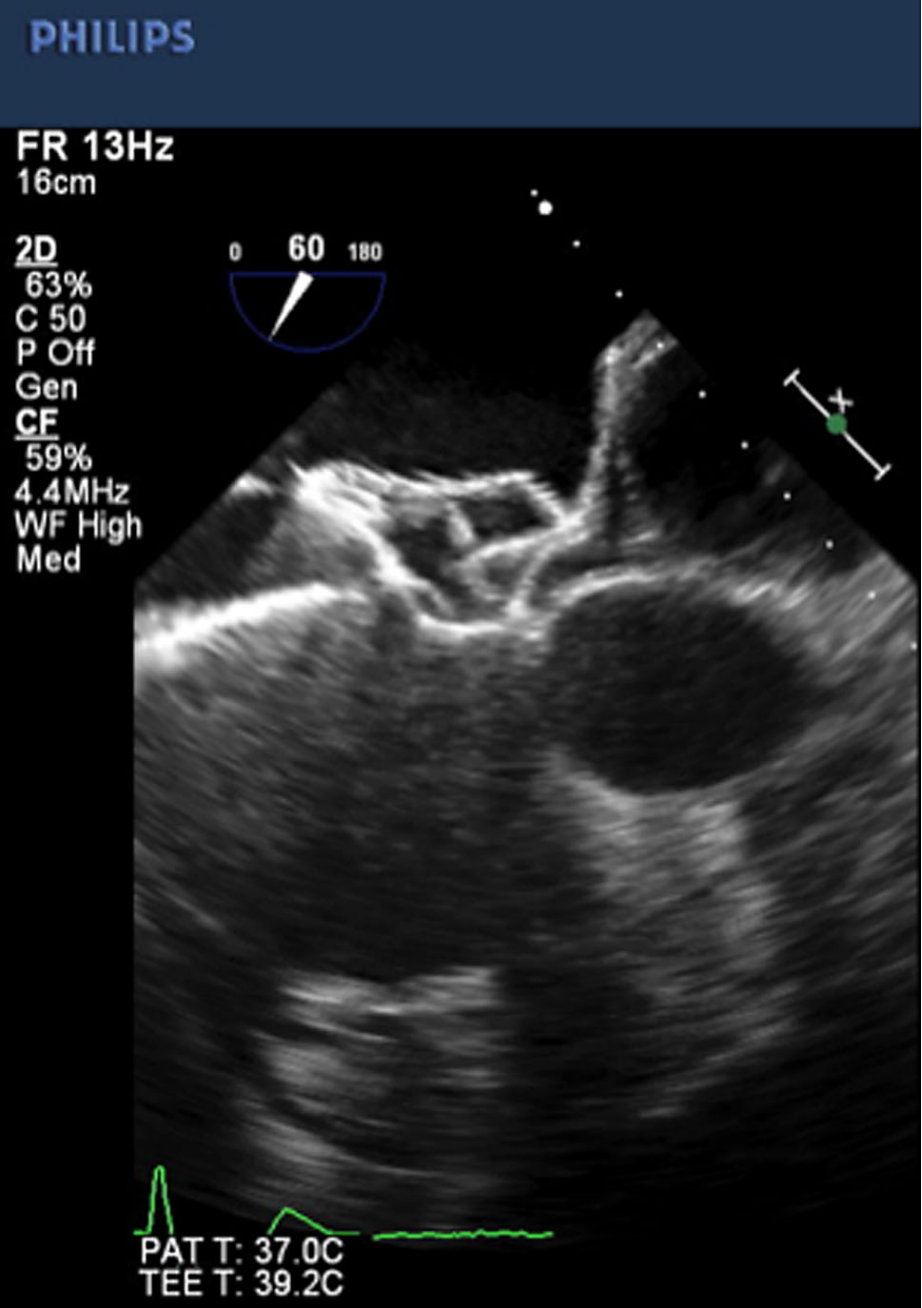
Transesophageal echocardiographic image after deploying the Figulla Flex II ASD occluder. The cable remains connected. A ball-shaped connector design allows a tilt of up to 50°, facilitating placement before release
